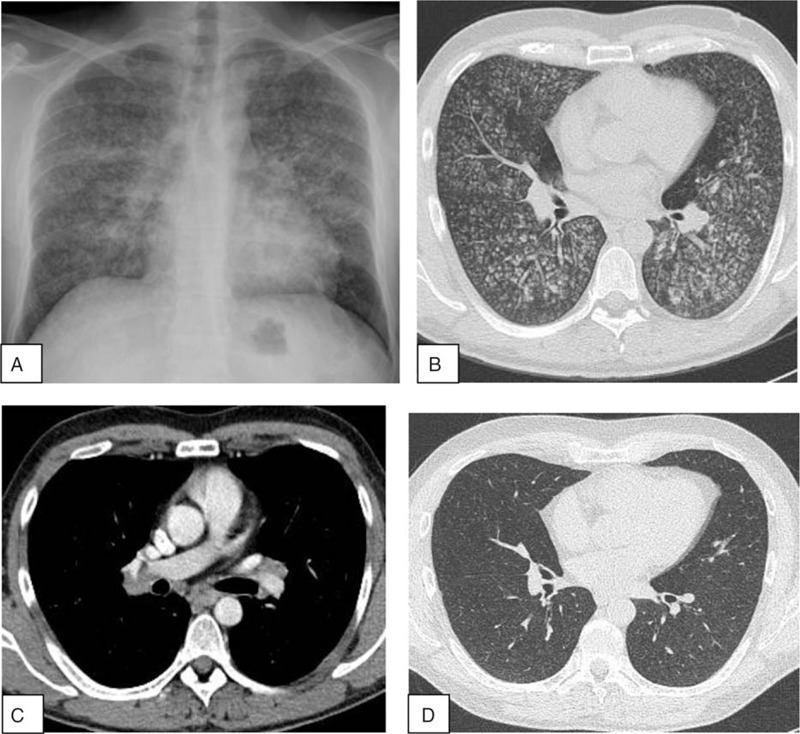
(A) Chest X-ray showing a micronodular pattern. (B) HRCT scan showing diffuse small centrilobular nodules. (C) Presence of interbronchial lymph nodes. (D) Improvement of miliary pattern after steroid therapy.
